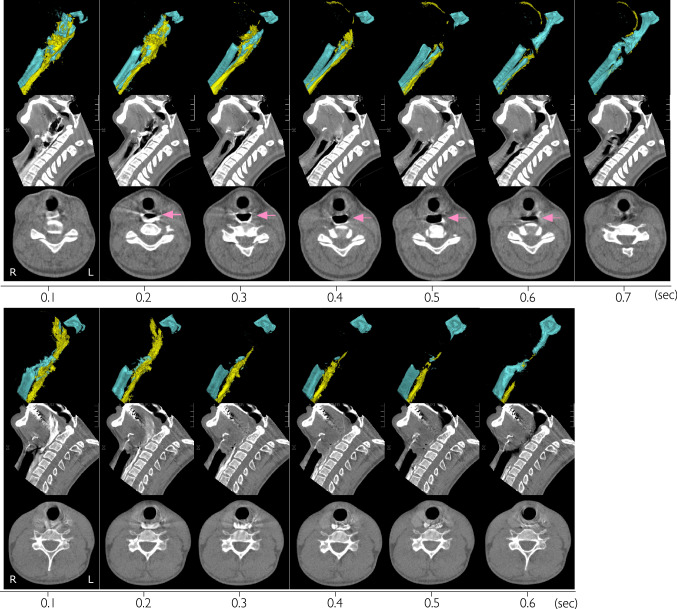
Lateral, mid-sagittal, axial views (upper, middle, and lower rows, respectively) of 3D-CT images during swallows of thin and thick liquid in representative subjects. Upper three rows are the images of thin liquid swallowing in a representative subject (37y, female). Lower three rows are the images of honey liquid swallowing in a representative subject (24y, male). In both upper and lower parts, first line is lateral 3D-CT images (yellow: bolus, blue: air column surface), second line is mid-sagittal sections, and third line is axial sections of UES. Arrows show annular flow. L: left R: right
